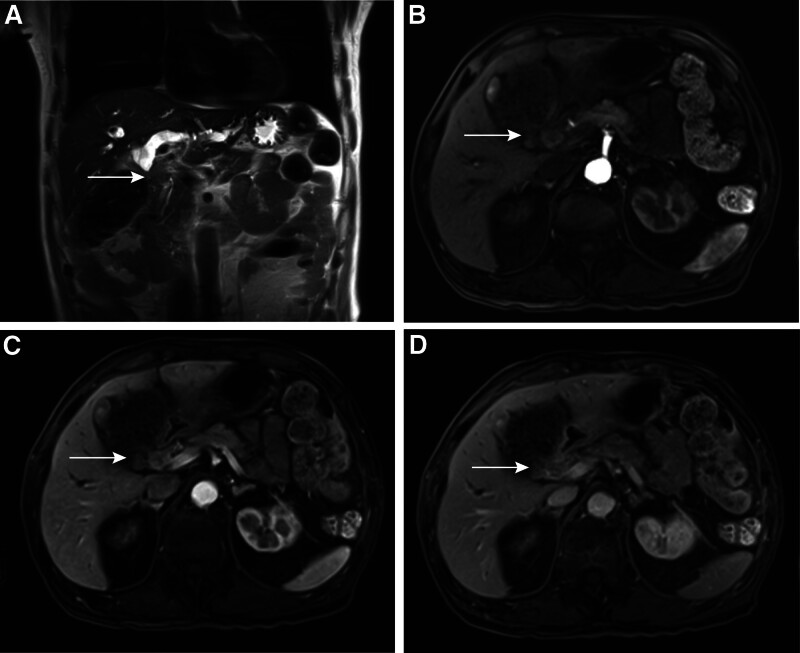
Enhanced abdominal MRI of the patient (preoperative). (A) The MRI shows significant dilation of the intrahepatic bile ducts and stenosis at the junction of the left and right hepatic ducts (indicated by the white arrows), whereas the distal bile ducts are not visible. Abdominal MRI in the arterial phase (B), portal venous phase (C), and delayed phase (D) reveals a space-occupying lesion in the hilar bile duct (The white arrow indicates enhancement of the hilar bile duct, with thickening of the duct wall). MRI = magnetic resonance imaging.
